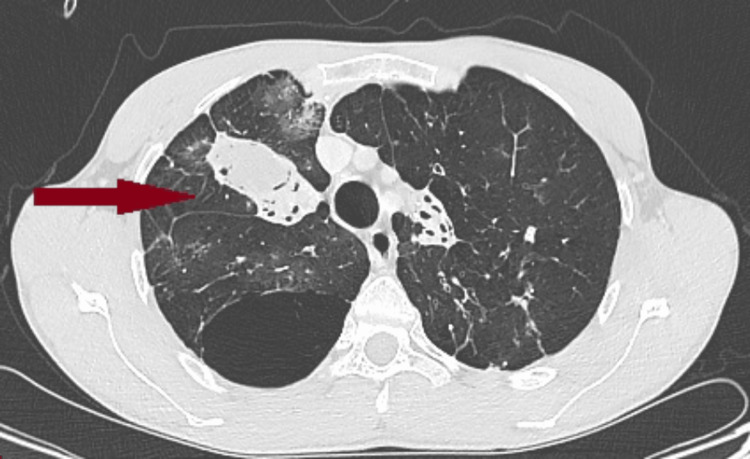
Transverse section of the preoperative thoracic CT scan showing a lesion in the right lung, involving both the upper and middle lobes (red arrow).
